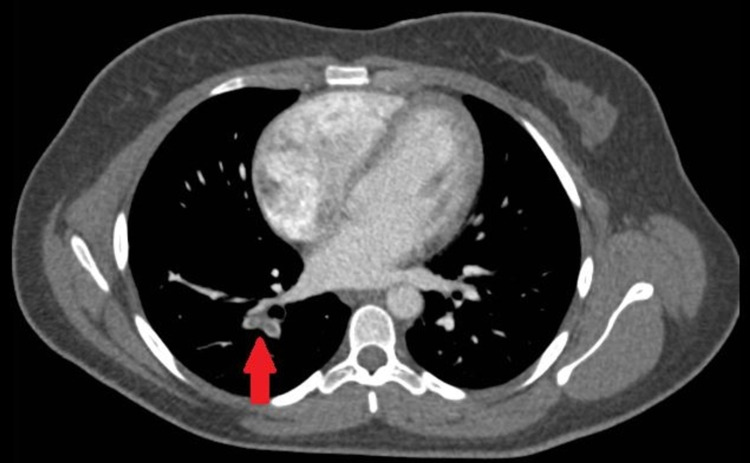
Axial CT imaging revealing pulmonary embolism in the right lower lobe segmental pulmonary arteries (arrow)
